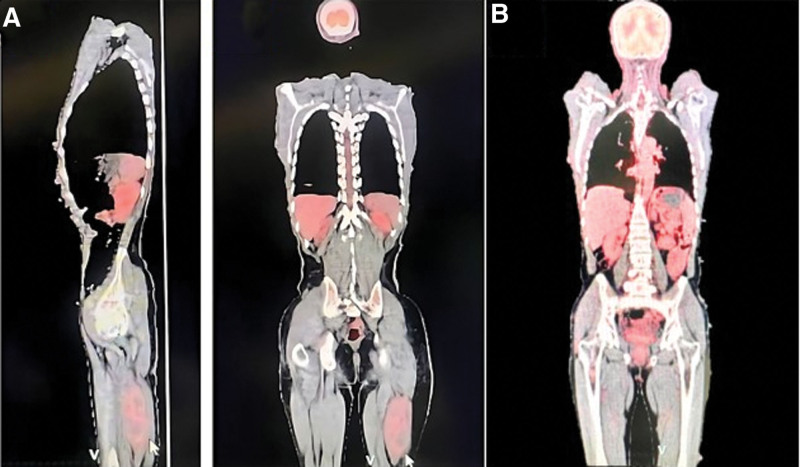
(A) FDG-PET scan showed a solid mass with enhanced metabolic activity in the left dorsal thigh and neurofibromas throughout the body under-skin. No metastases was noted. (B) After 10 yr follow up FDG-PET scan showed no metastasis. FDG-PET = fluorodeoxyglucose-positron emission tomography.
